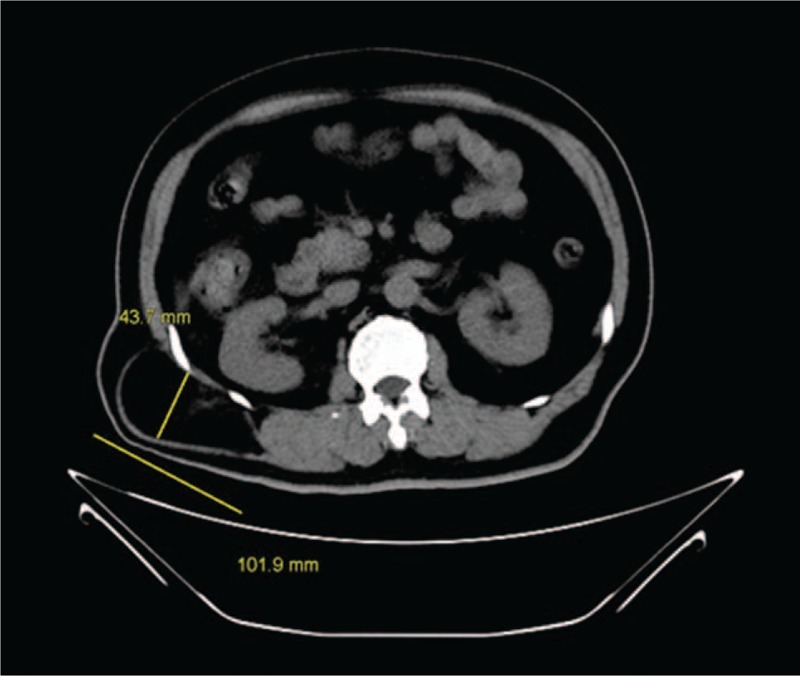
Transverse section of a CT scan imaging showing the primary right lumbar hernia. CT = computed tomography.
